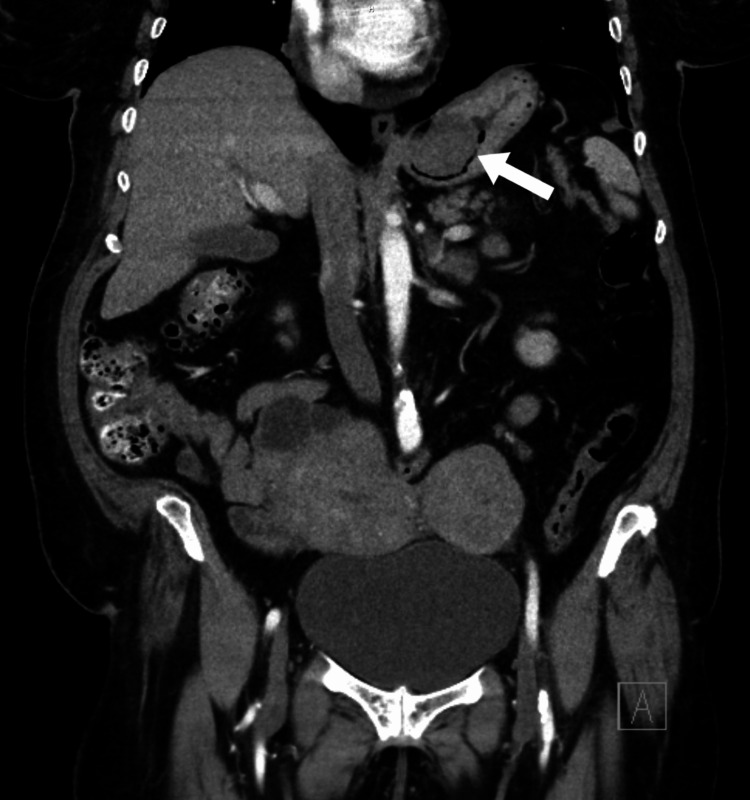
Computed tomography with contrast in coronal plane. Gastric leiomyoma is indicated by the arrow.
